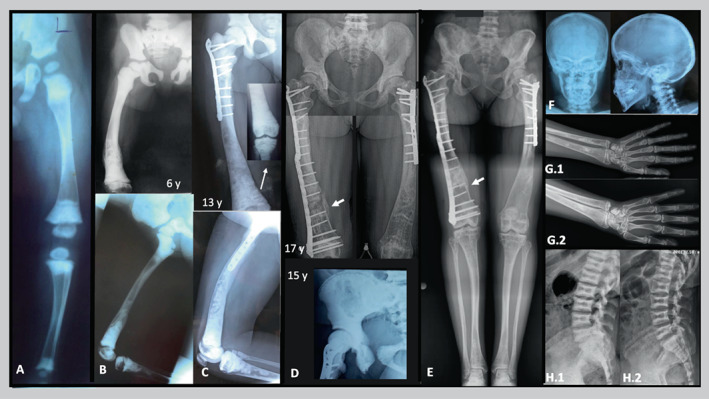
Patient 1. Evolution of radiographic findings at increasing age. (*A*) In early childhood the pelvis and metaphyses and epiphyses of the lower extremities are densely osteosclerotic. (*B*) At age 6 years, osteosclerosis is more diffuse and also apparent in the lower spine. Right femoral tubulation error and transverse healing fracture are apparent distally. (*C*) At age 13 years, the right femur shows coxa vara, diffuse but patchy osteosclerosis, loss of cortical and medullary distinction, plate and screw fixation of a proximal fracture, removal of distal femoral hardware, and metaphyseal and distal diaphyseal expansion. The right knee is shown as an inset (arrow). (*D*) At age 15 years, the pelvis and femoral heads are densely osteosclerotic, but less so centrally, giving a “bone‐in‐bone” appearance. At age 17 years, the femora are becoming osteopenic but with coarse trabeculation and focal osteosclerosis, have hardware fixation of a fracture, an unbridged distal right fracture (arrow) that persisted at age 18 years (not shown), and distal metadiaphyseal expansion. (*E*) At age 23 years, standing radiograph shows improvements including the pelvis and lower extremities with decreased osteosclerosis and tubulation error, improved mineralization, and a solidly bridged fracture (arrow). There is expanded metadiaphyseal bone in the distal femurs and tibias, overall osteopenia, focal osteosclerosis, thick trabeculae, and bowing. (*F*) Anteroposterior and lateral views of the skull at age 12 years reveal mild diploic thickening and diffuse osteosclerosis that includes the base, orbits, facial bones, and cervical spine. (*G.1*) At age 17 years, the distal forearms have metadiaphyseal expansion and focal osteosclerosis. The carpals show peripheral osteosclerotic ringing, metacarpals and phalanges patchy osteosclerosis, and metacarpals some error of tubulation. (*G.2*) At age 23 years; the tubulation errors are more prominent, but with improvement in the patchy osteosclerosis. (*H.1*) Between ages 17‐1/2 years and 22 years (*H.2*), the vertebral endplate sclerosis (“sandwich vertebrae”) is less, matching the BMD *Z*‐scores that decreased from +8.7 to +7. The vertebral body flattening and decreasing osteosclerosis apparent in the later views of the pelvis were little changed (not shown).
